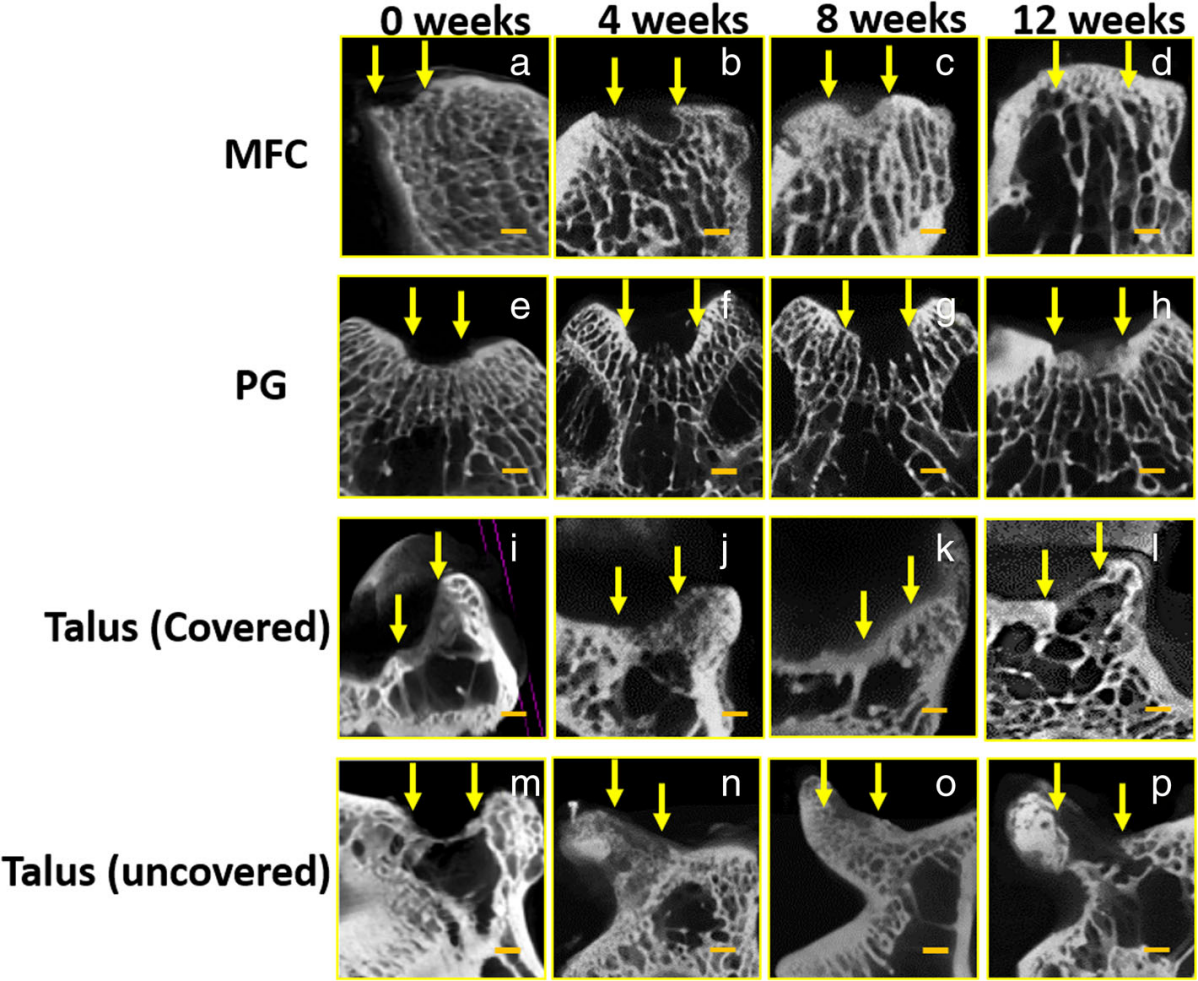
Computed tomography findings of osteochondral defects of the medial femoral condyle (MFC) (a–d), patellar groove (PG) (e–h), covered talus (i–l), and uncovered talus (m–p) at 0, 4, 8, and 12 weeks after surgery. *Arrows* indicate the osteochondral defect. *Scale bar*, 1000 μm
